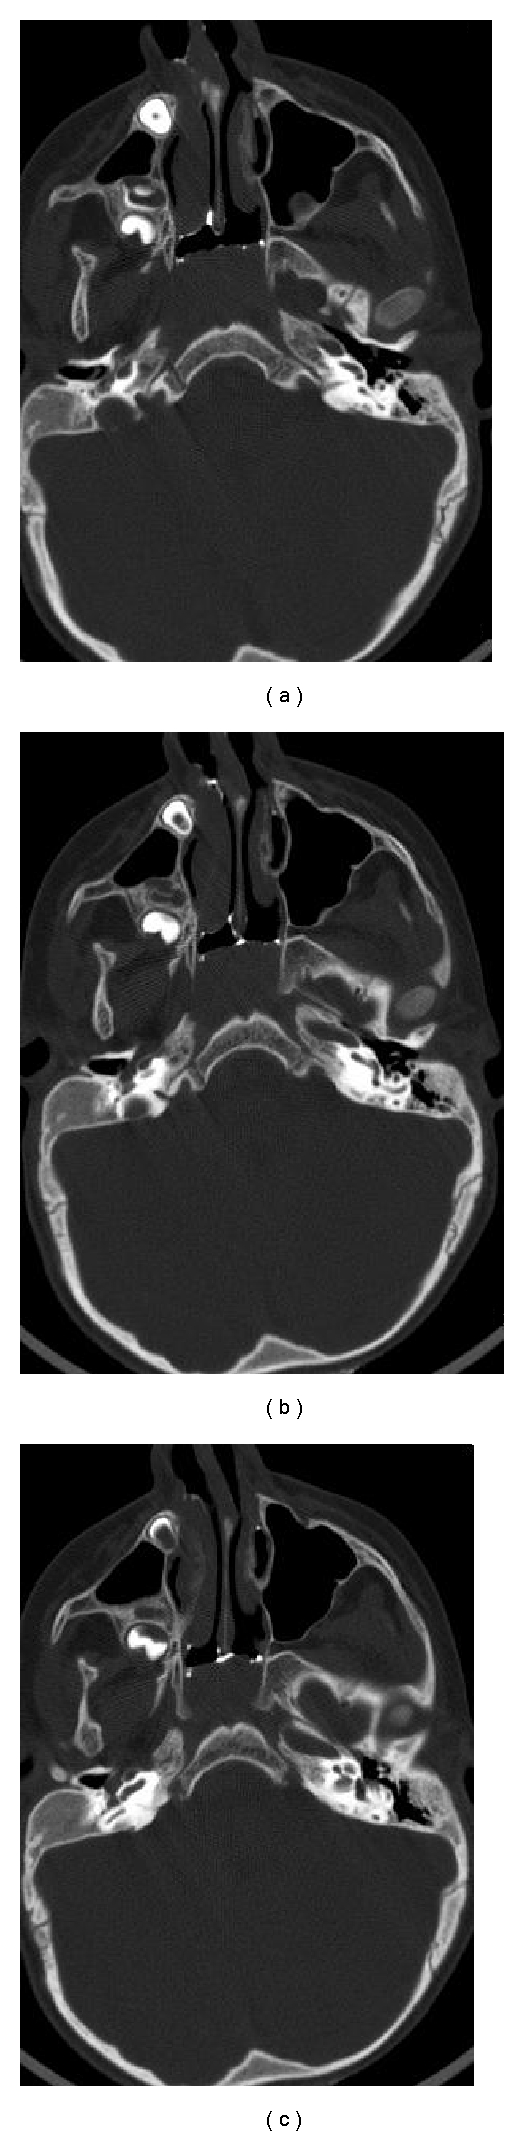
Axial CT with contrast showing hypertrophy of the inferior turbinate and patent choana.
